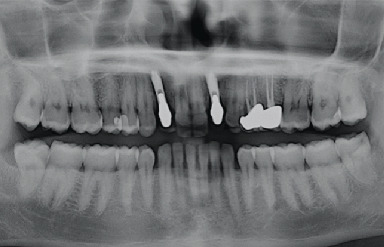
Orthopantomography at 20 years.
